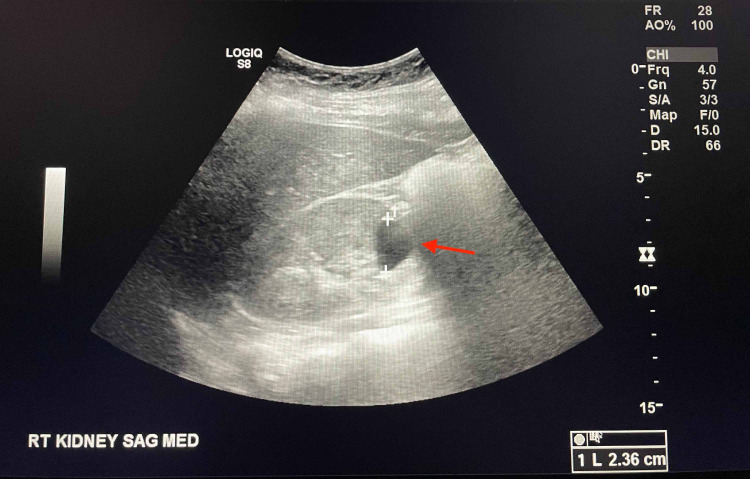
Sagittal view: ultrasonography of right kidney showing hypoechoic cystic structure (red arrow)
